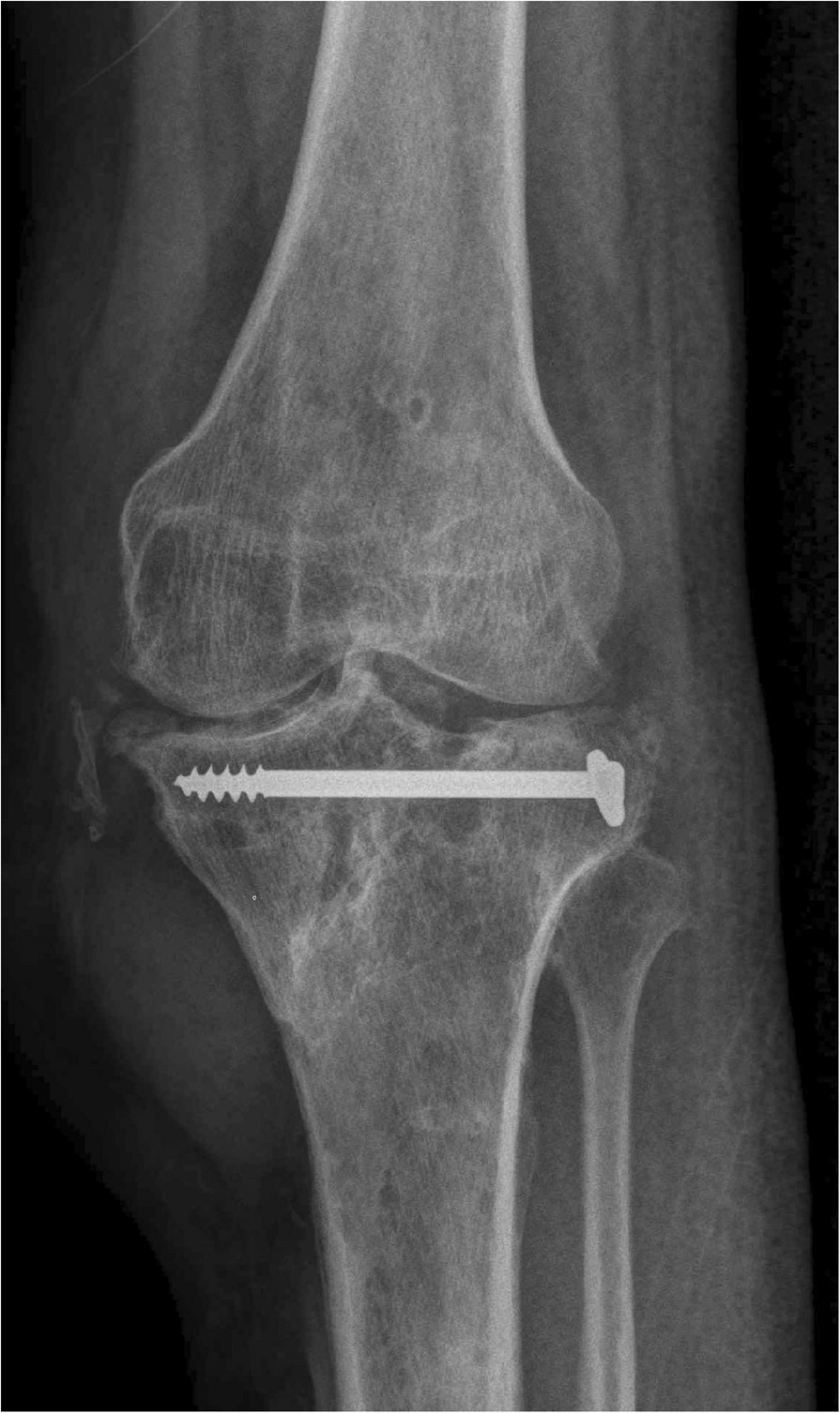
Radiograph (antero-posterior view) of our patient’s left knee and leg, taken one year after surgery, showing the proximal tibia fracture united.
